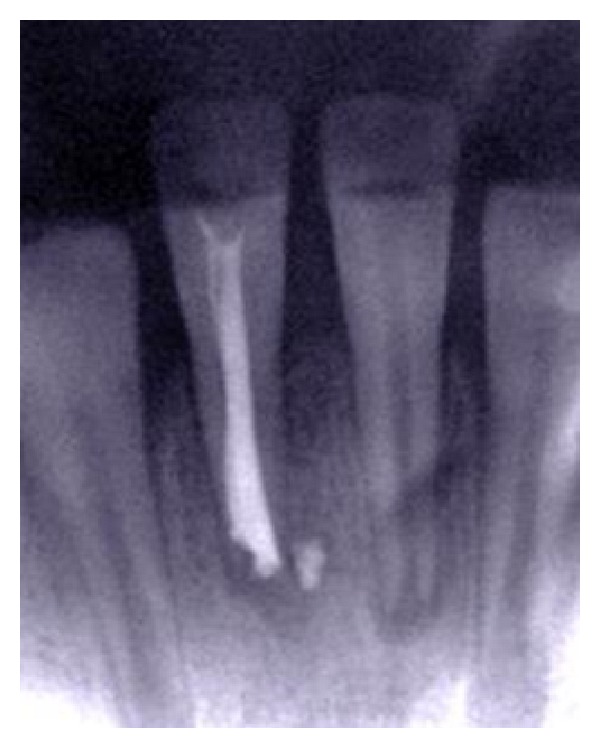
Three-month follow-up radiograph.
